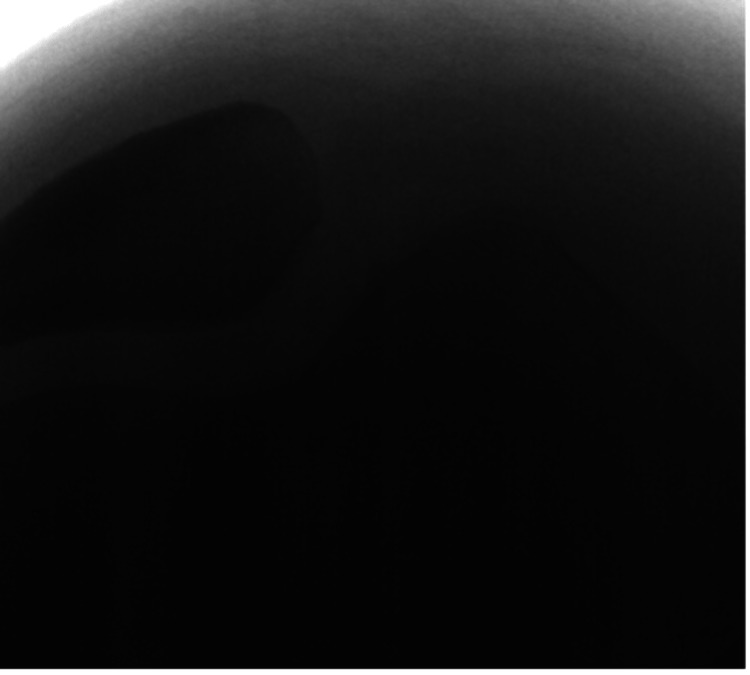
Intra-reduction fluoroscopy sunrise radiograph of the left knee confirmed a successfully reduced patella
